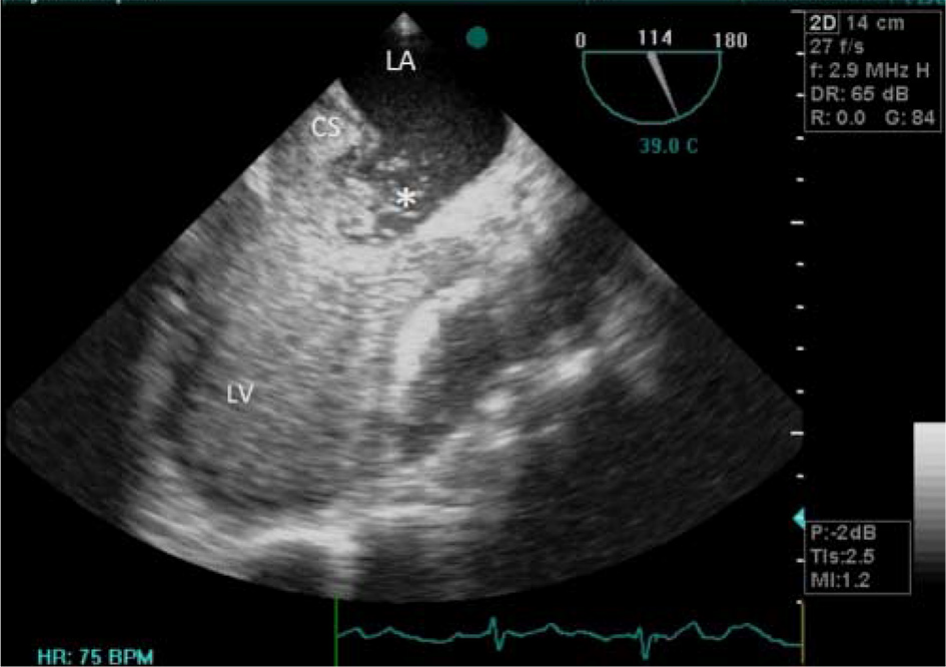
Transesophageal Echocardiography at 115 Degree Showing Large Defect (Asterisks) in Mid Part of Coronary Sinus With Significant Left to Right Shunt (Negative Contrast) During Agitated Saline Injection CS, coronary sinus; LA, left atrium; LV, left ventricle.
